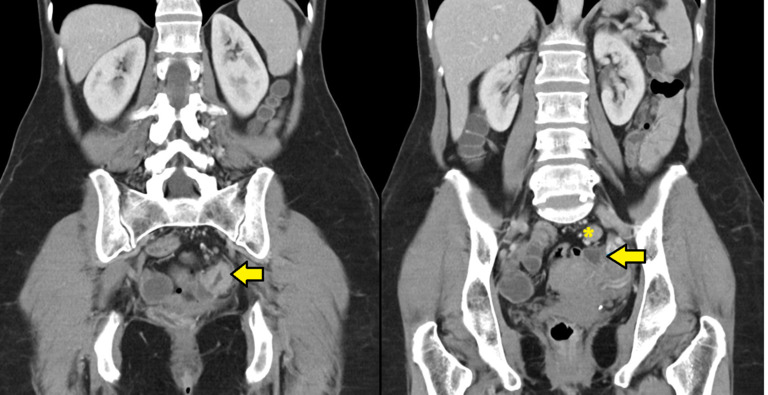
A coronal computed tomography image shows circumferential parietal thickening causing luminal stenosis (arrow). Regional lymph nodes are also observed, the largest (asterisk) of 16 mm for the long axis diameter and suspicious for malignancy.
